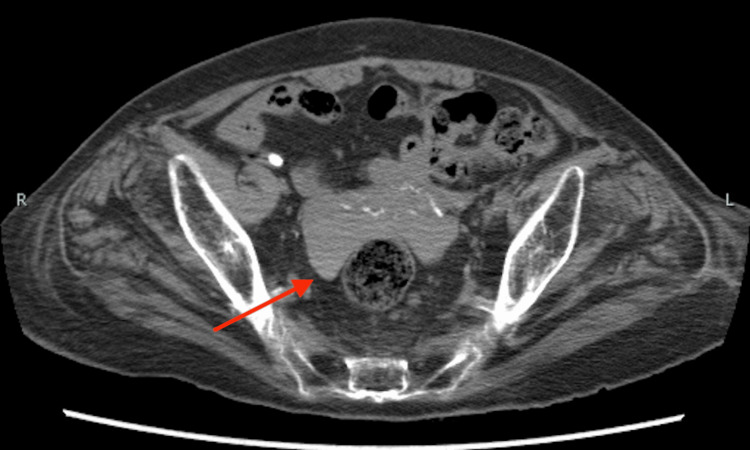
CT image (pelvic cavity) CT image demonstrating blood accumulation in the pelvic cavity, suggestive of intra-abdominal hemorrhage.
